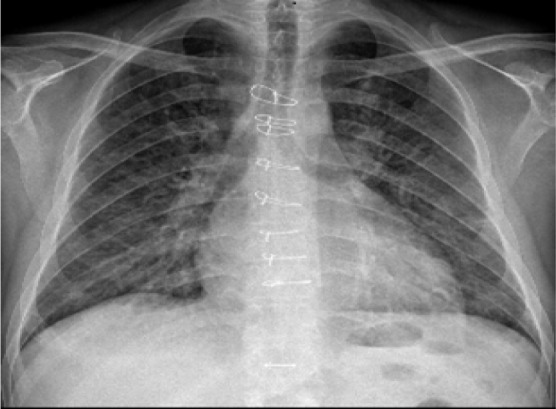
Chest radiography of patient
